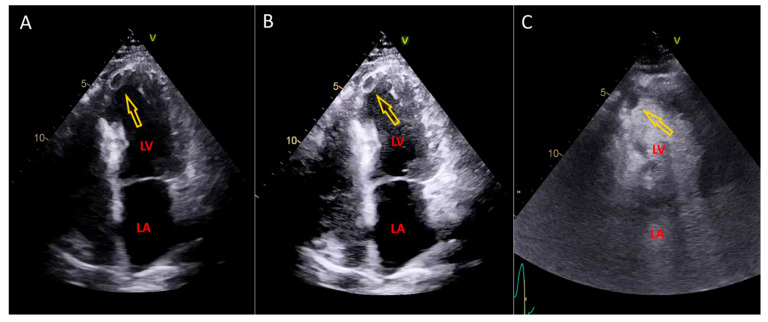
Echocardiography images depicting thrombus in the left ventricle obtained by conventional echocardiography, after postprocessing with LVTDM, and contrast echo. (A). Conventional echocardiography image showing suspicion of thrombus in the apex of the left ventricle [arrow], requiring confirmation by additional methods. (B). Image obtained after postprocessing with LVTDM, providing improved visualization of thrombus in the left ventricle [arrow]. (C). Contrast echocardiography image following injection of SonoVue. A filling defect in the apex of the left ventricle is consistent with thrombus [arrow]. Abbreviations: LV—left ventricle, LA—left atrium.
